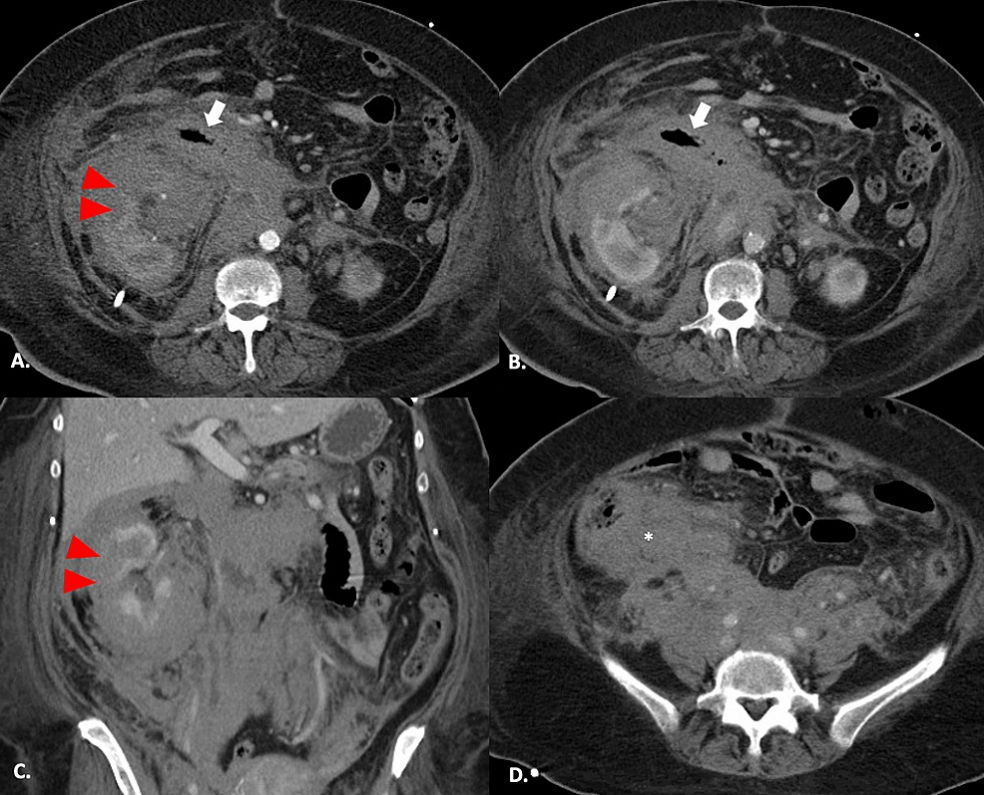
Follow-up CT scan acquired one month after the initial presentation. Axial arterial and portal enhanced CT images (A and B) demonstrate an increase of its extension. The densification now involves circumferentially the duodenum (white arrows) reducing its lumen. Portal venous phase (C) depicts markedly irregularity of the right kidney (red arrowheads) and encasement of the renal vessels. Axial portal venous phase (D) showing peri-cecum densification with loss of the fat cleavage plan (white asterisk). There wasn't significant variability of attenuation compared to the initial evaluation. These findings ruled out the hypothesis of hematoma, and strongly suggest a lymphoproliferative disorder.
